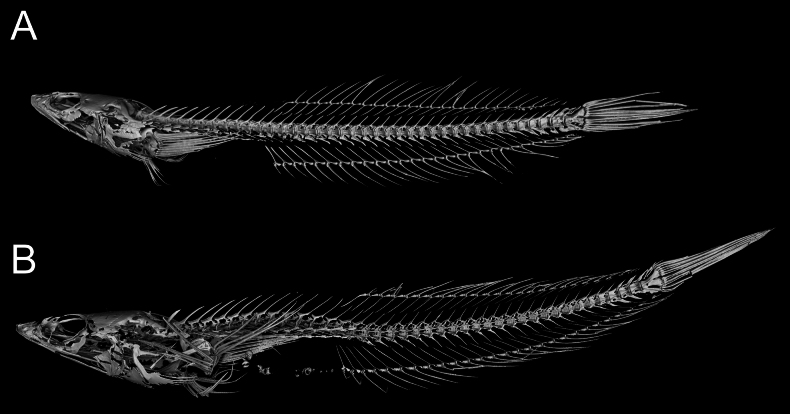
Radiographs of *Limnichthys* species using CT-scan A*Limnichthyskoreanus* sp. nov., holotype, MABIK PI00060703 (PKU 63120), 37.3 mm SL, South Korea B*Limnichthysfasciatus*, syntype, AMS I.5858, 43 mm SL, preserved in Australian Museum.
